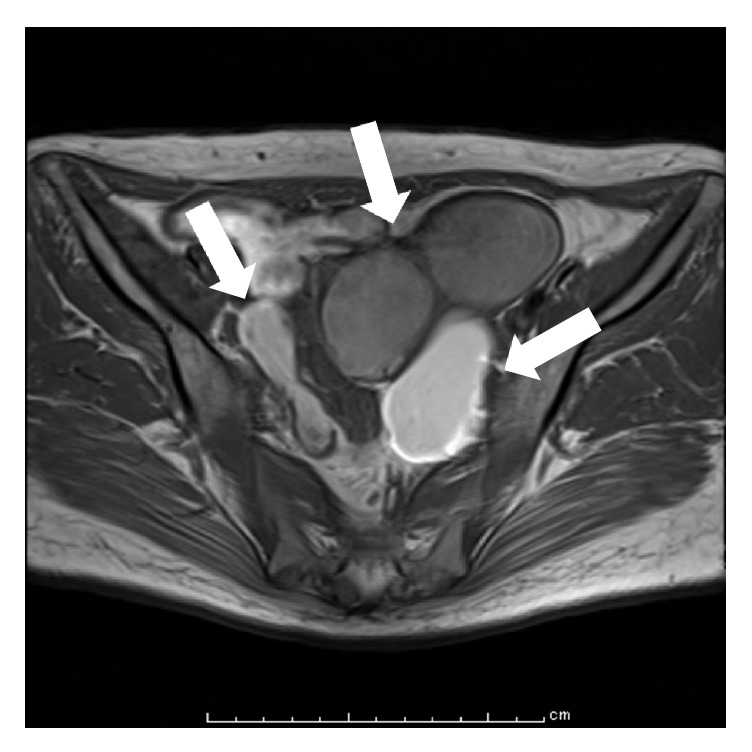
Pretreatment magnetic resonance imaging of our patient with endometriosis. Axial T1-weighted imaging at the first consultation. Two ovarian tumors (tumor size: right < left) show high-intensity signals (arrows), and the internal structure shows a blood-resistant component.
